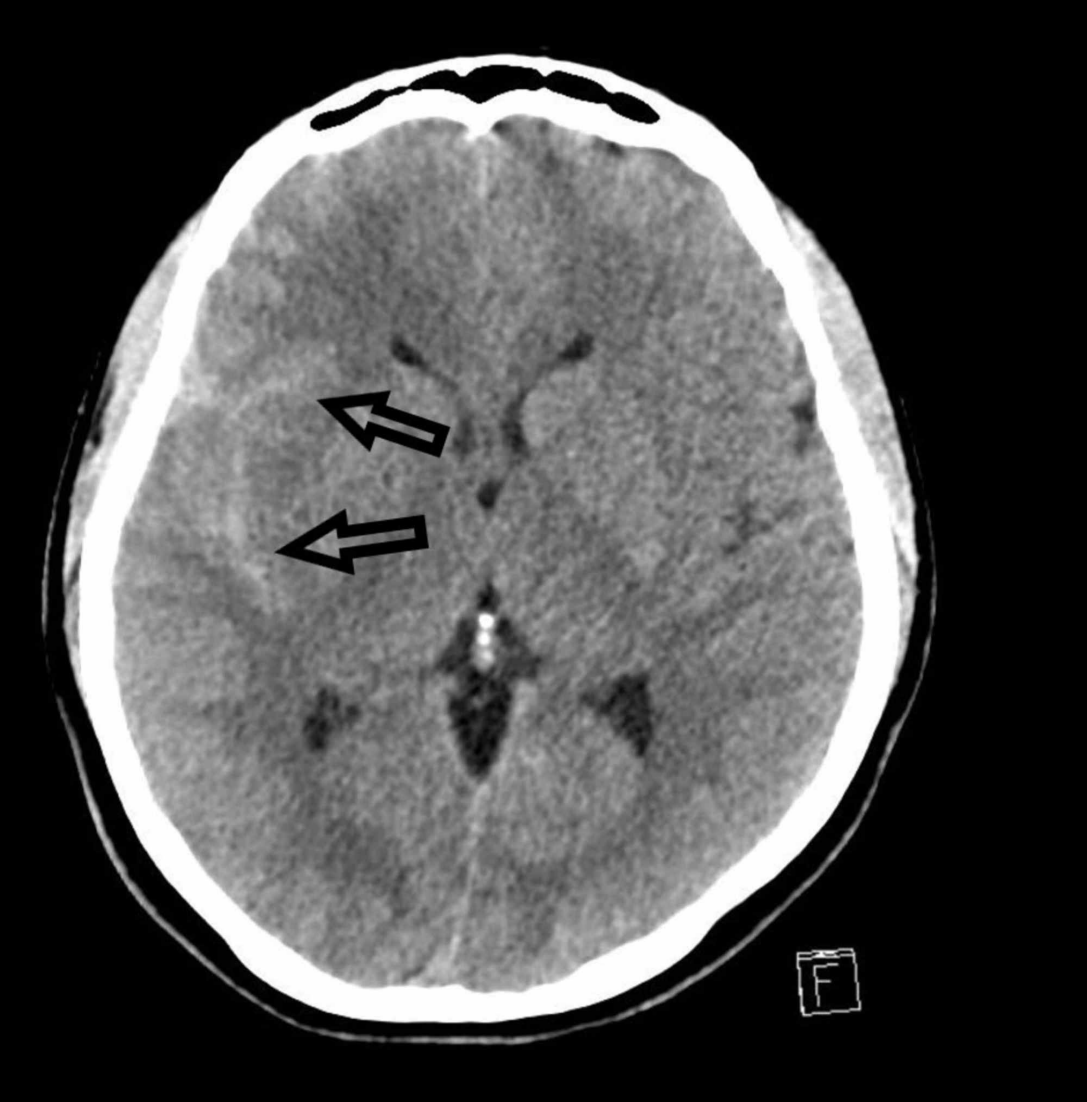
CT of the brain: axial view A CT of the brain showing blood along the Sylvian fissure. This image is more cephalad than the image shown in Figure 1.
